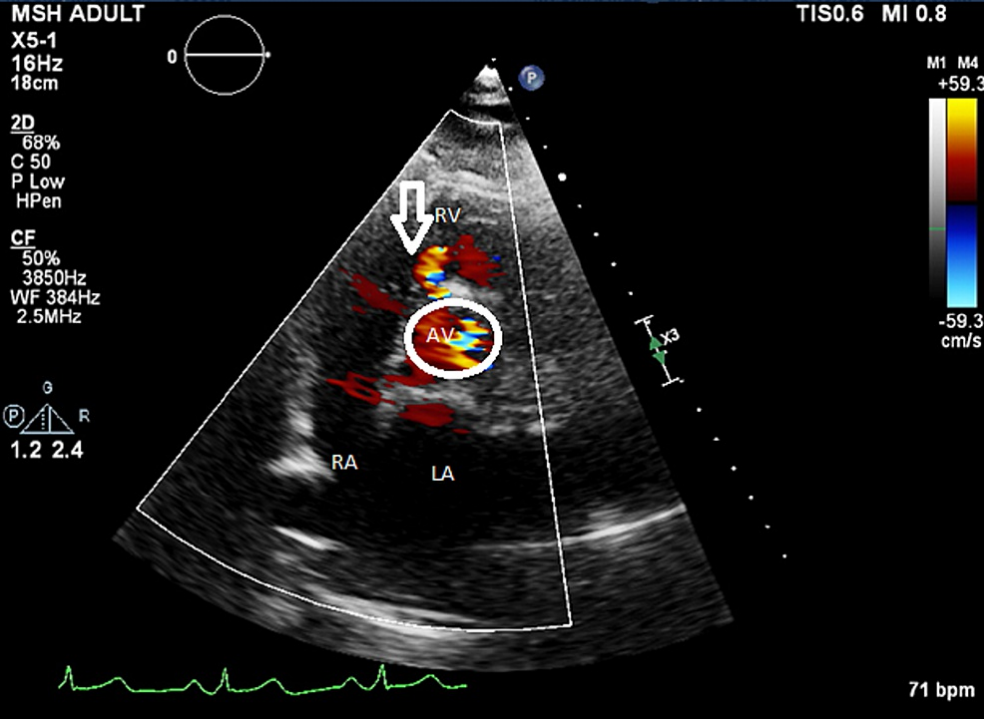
Transesophageal echocardiogram in the aortic valve short-axis view with the visible VSD jet at approximately 11 O’clock position VSD: ventricular septal defect; RA: right atrium; LA: left atrium; AV: aortic valve (circle) The arrow indicates the 11 O’clock position.
